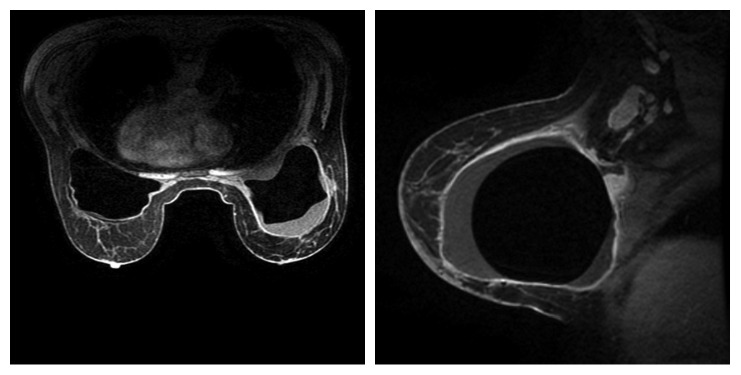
MRI at diagnosis showing fluid surrounding right breast implant, enlarged right axillary lymph node.
